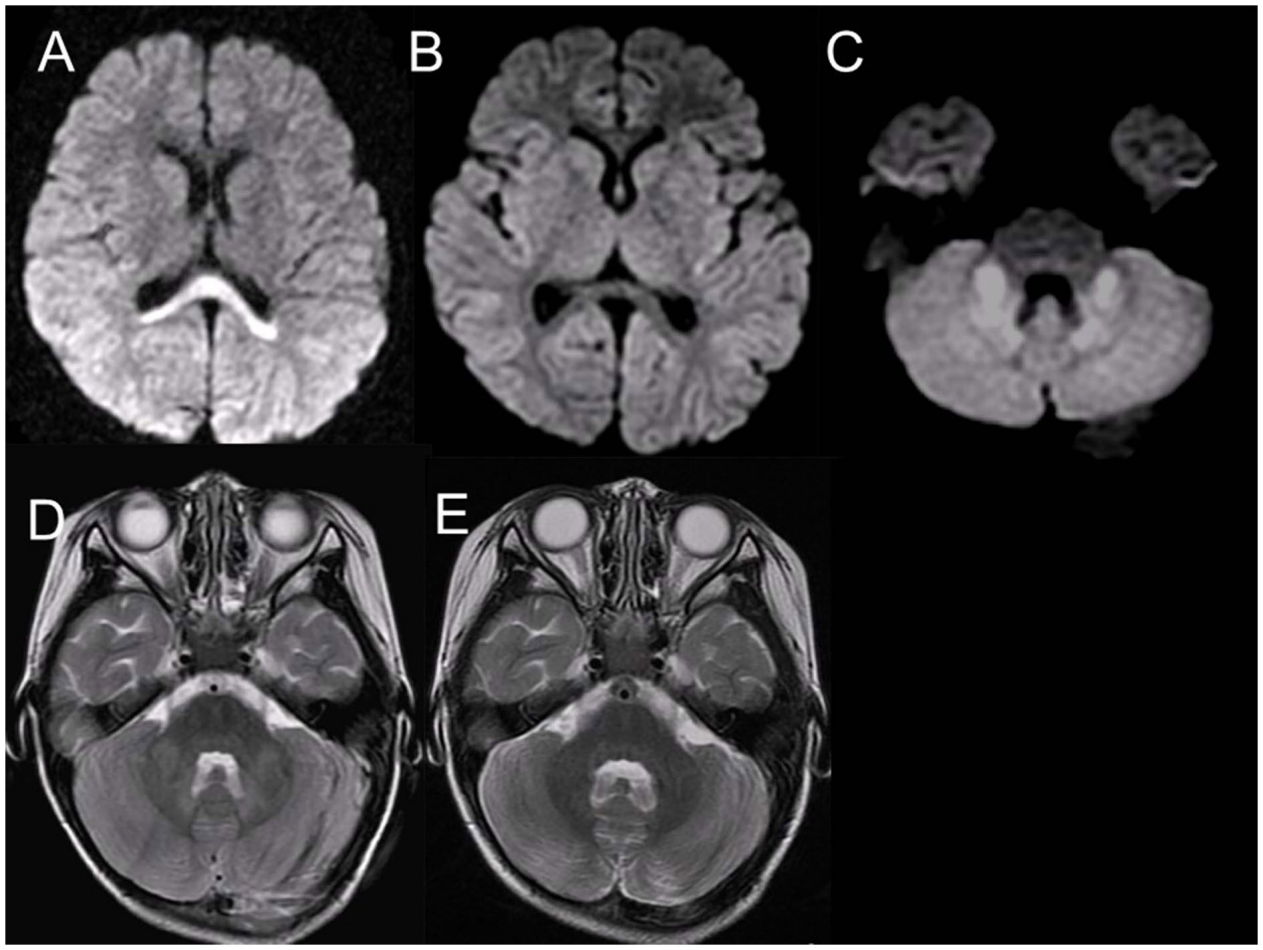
MRI of 3-year-old girl with cerebellitis associated with rotavirus. DWI on day 4 (A) shows a splenial lesion compatible with type 1 MERS, which disappeared on day 6 (B). DWI on day 6 shows abnormally reduced diffusion in the bilateral middle cerebellar peduncles and nuclei (C). T2WI also shows mild hyperintensity in the cerebellar cortex in addition to the middle cerebellar peduncle and nuclear lesions (D). MRI on day 65 shows an almost normal cerebellum, other than mild atrophy (E) (from Takanashi et al., 2010b with permission). MRI, magnetic resonance imaging; DWI, diffusion-weighted imaging; MERS, clinically mild encephalitis/encephalopathy with reversible splenial lesions; T2WI, T2-weighted imaging.
